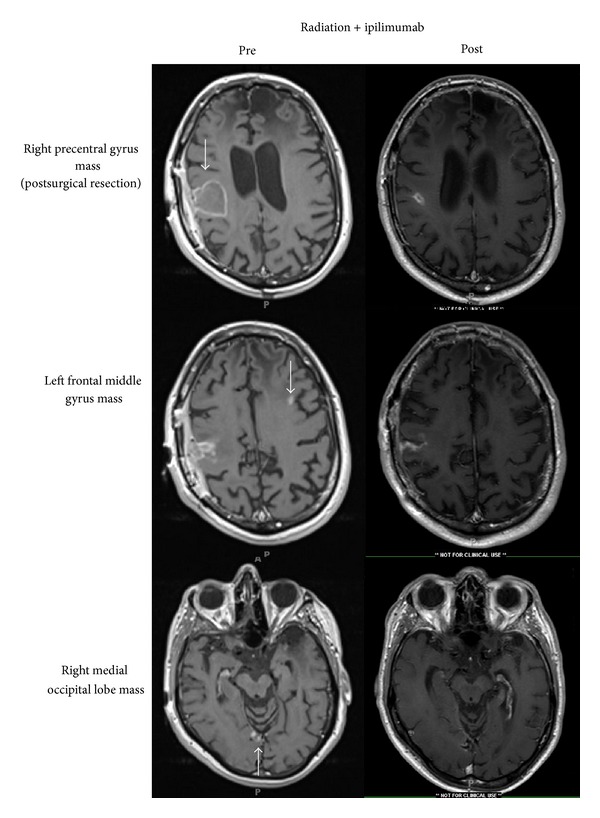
T1-postcontrast MRI showing pretreatment (left column) and 8-month posttreatment (right column) MR imaging of melanoma brain metastases treated with SRS and ipilimumab.
